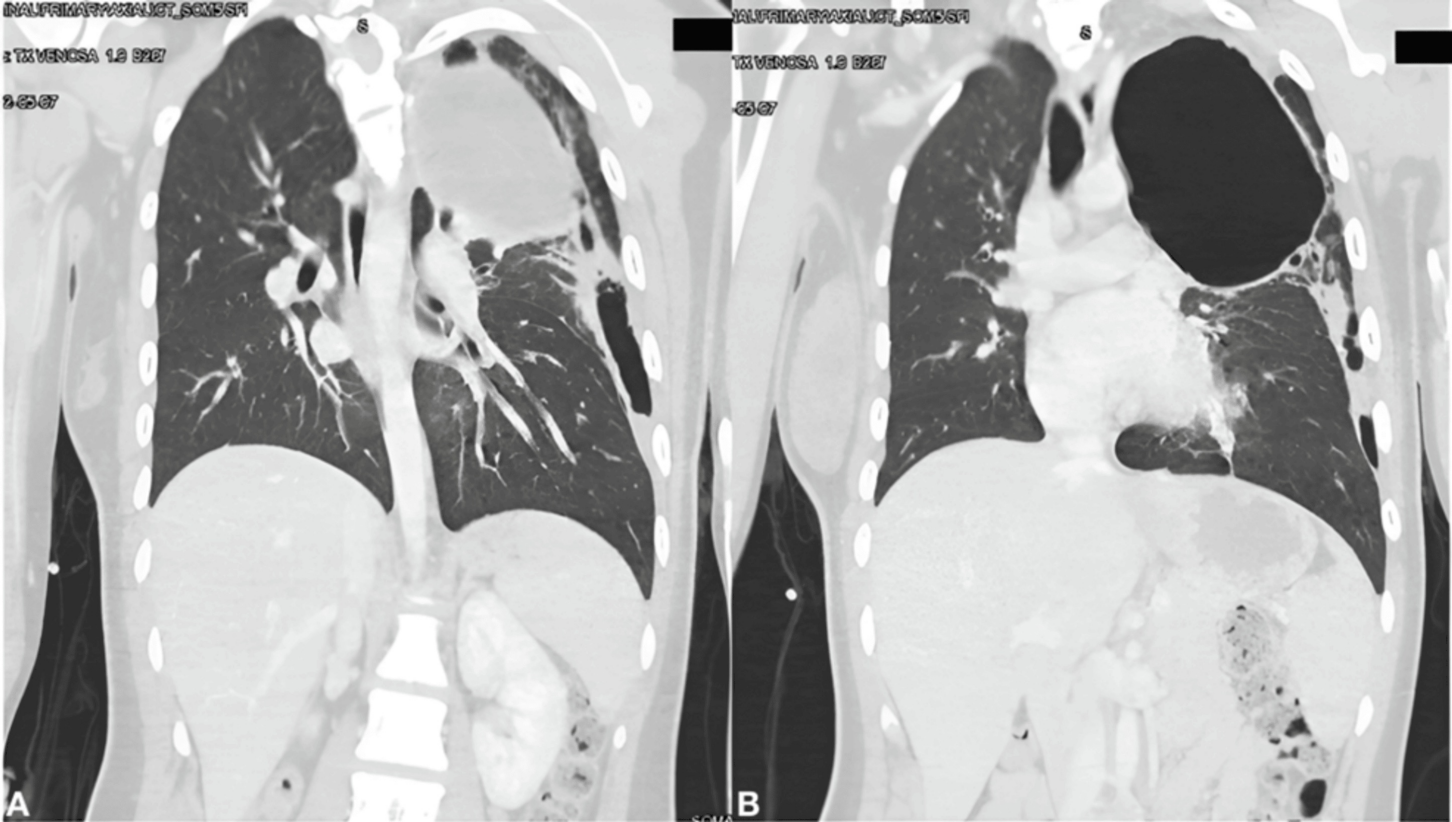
Preoperative coronal CT scan (A) Cystic lesion of 7 cm in diameter with liquid content, without communication to the airway. (B) Cavitated lesion identified laterally and inferiorly to the cystic mass.
